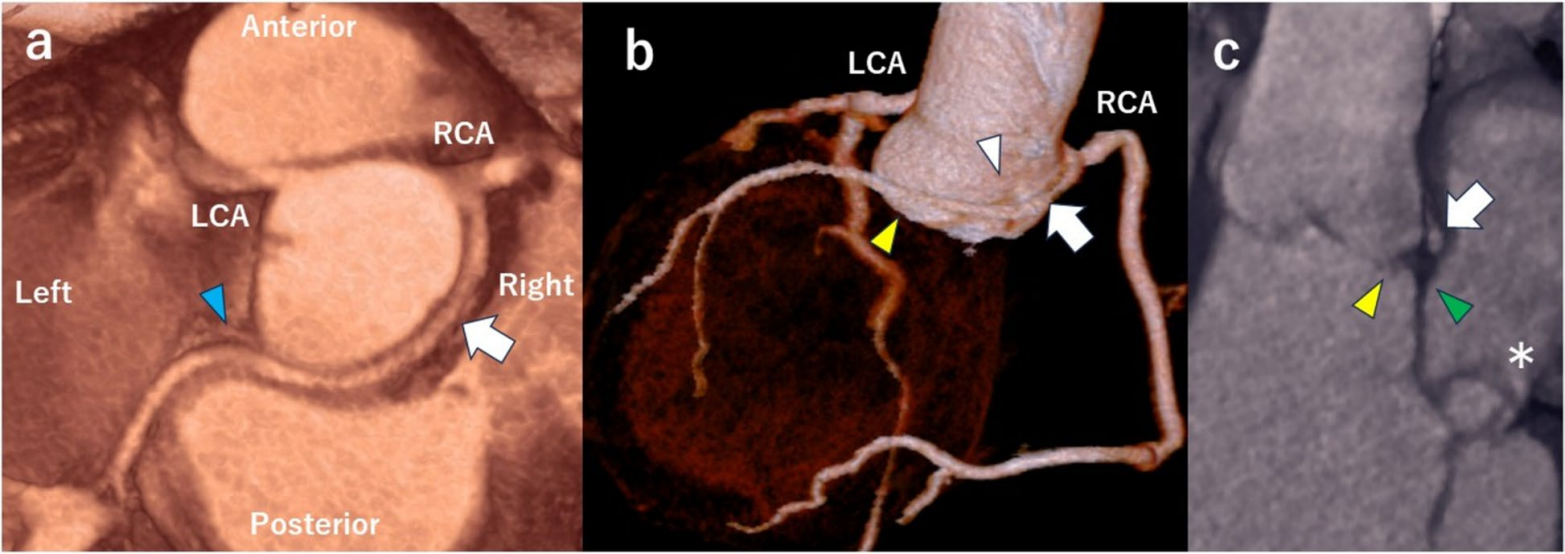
Preoperative computed tomography showing that the left circumflex coronary artery (white arrow) originated from the right coronary artery (a and b). It traversed the commissures of the right and noncoronary cusps of the aortic valve (white arrowhead) (b) and extended between the aorta and the right and left atria (a and b). The artery then continued to the right fibrous trigone (blue arrowhead), reaching the posterior atrioventricular groove (a). Between the aorta and the left atrium, it passed slightly above the aortic valve annulus (yellow arrowhead) and the mitral valve annulus (green arrowhead) (b and c). Additionally, an anterior mitral valve aneurysm (asterisk) was observed (c). *LCA*, left coronary artery; *RCA*, right coronary artery
